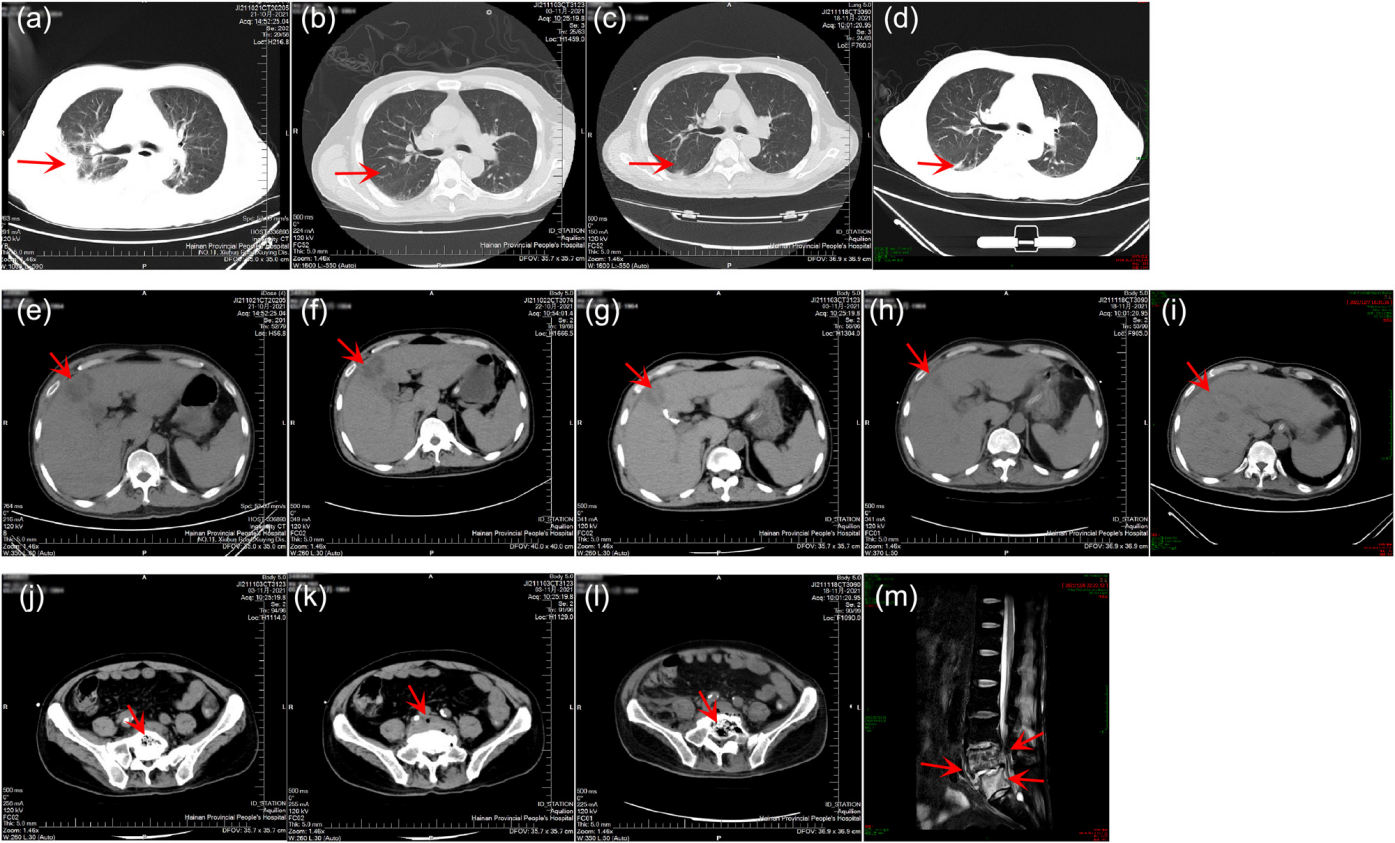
Imaging test results of the patient. (a)–(d) Lung CT results on October 21 (Day 1), November 3 (Day 14), November 18 (Day 29), and December 7 (Day 48), respectively. (e)–(i) Liver CT results on October 21 (Day 1), October 22 (Day 2), November 3 (Day 14), November 18 (Day 29), and December 7 (Day 48), respectively. (j) and (k) Abdominal CT results on November 3 (Day 14). (l) Abdominal CT results on November 18 (Day 29). (m) Lumbar MRI results on December 8 (Day 49).
